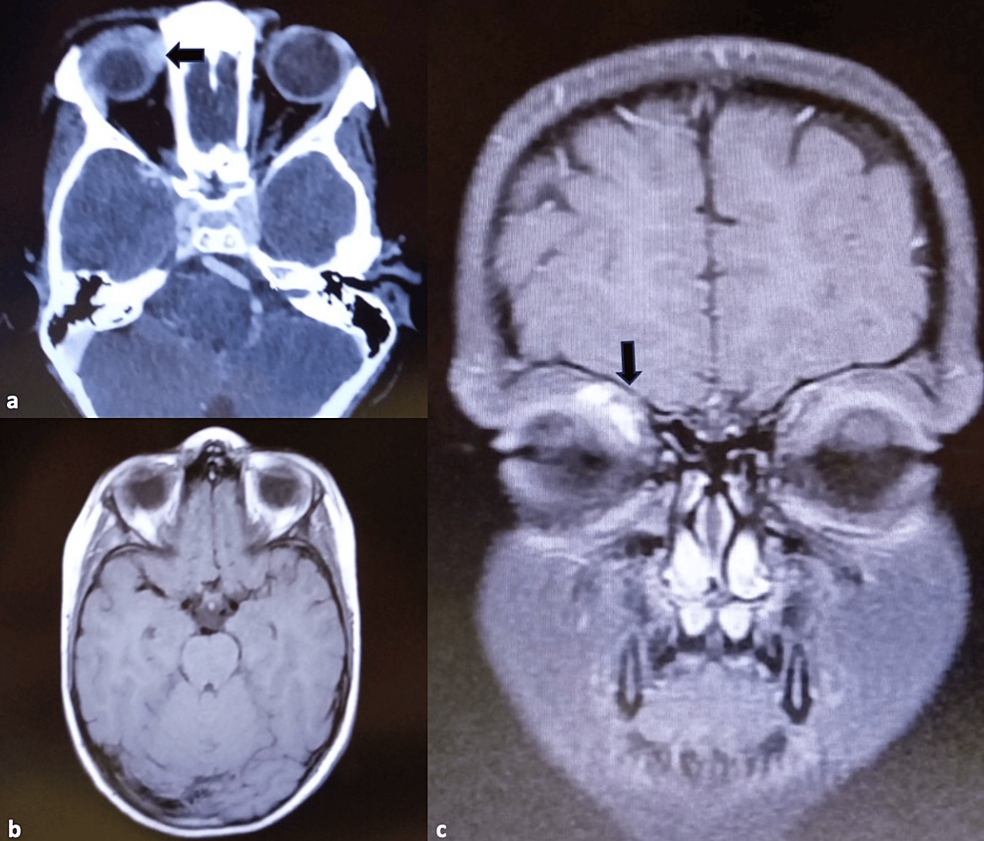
MRI of the brain and orbits. The residual tumor in the right orbit is shown with black arrows in (a) and (c). No metastasis was detected on the brain MRI (b).
